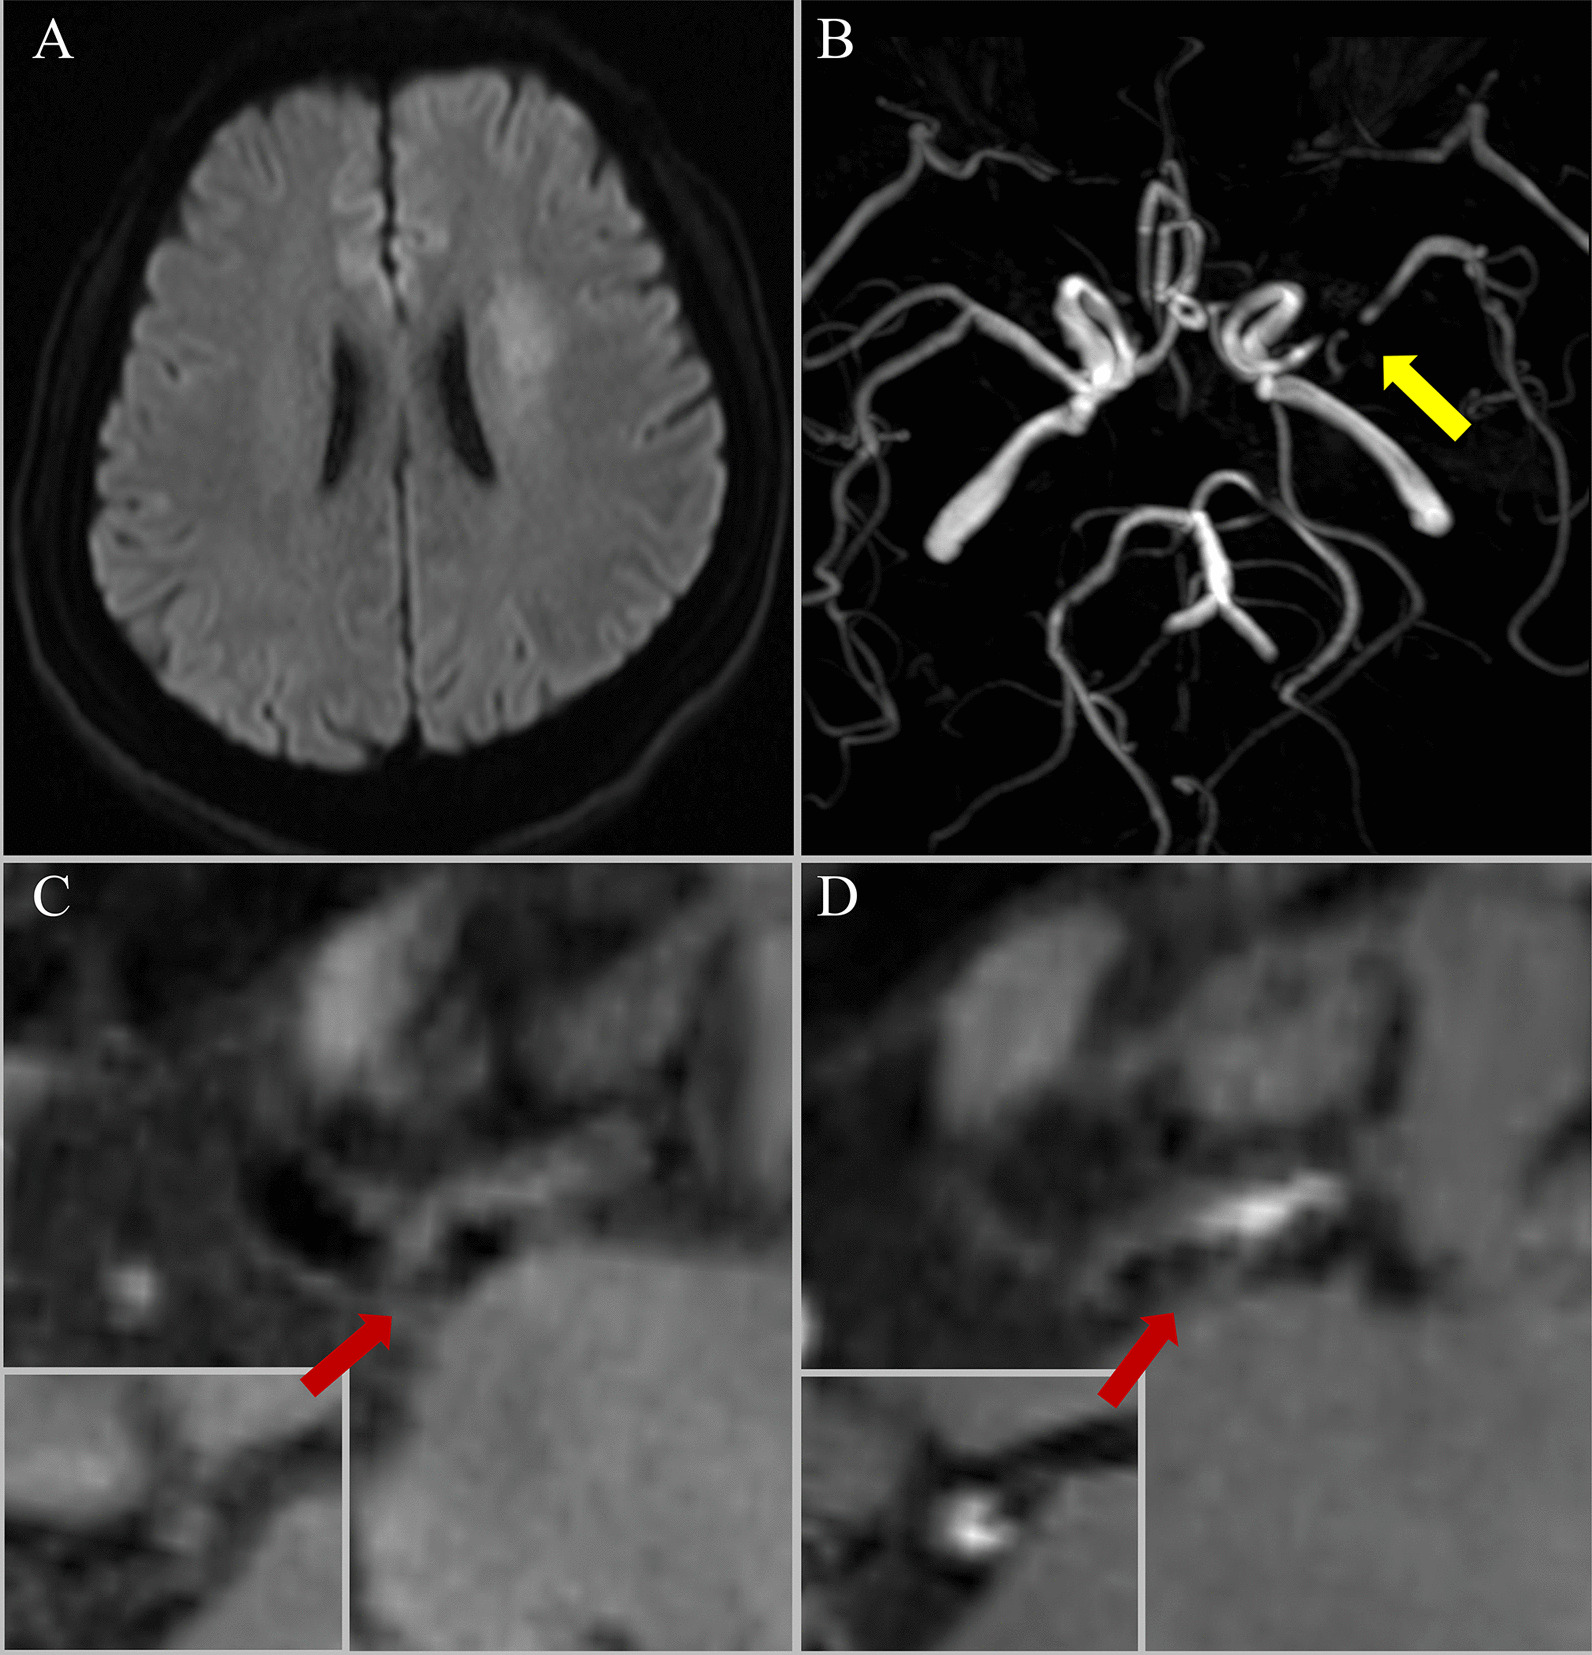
Representative case of a 58-year-old woman who was diagnosed with stroke before performing the CMR scan. A Diffusion weighted imaging (DWI) showed high signal intensity lesions on the left centrum ovale. B time of flight cardiovascular magnetic resonance angiography (TOF-CMRA) showed severe stenosis (yellow arrow) in the left middle cerebral artery (MCA) M1. C, D Curved multiplanar reconstructions of the C pre-contrast and D post-contrast HR-VWI showed a focal plaque in the left MCA with severe stenosis (stenosis ratio = 87.25%), irregular plaque surface, and grade 2 enhancement (plaque enhancement ratio = 3.13)
